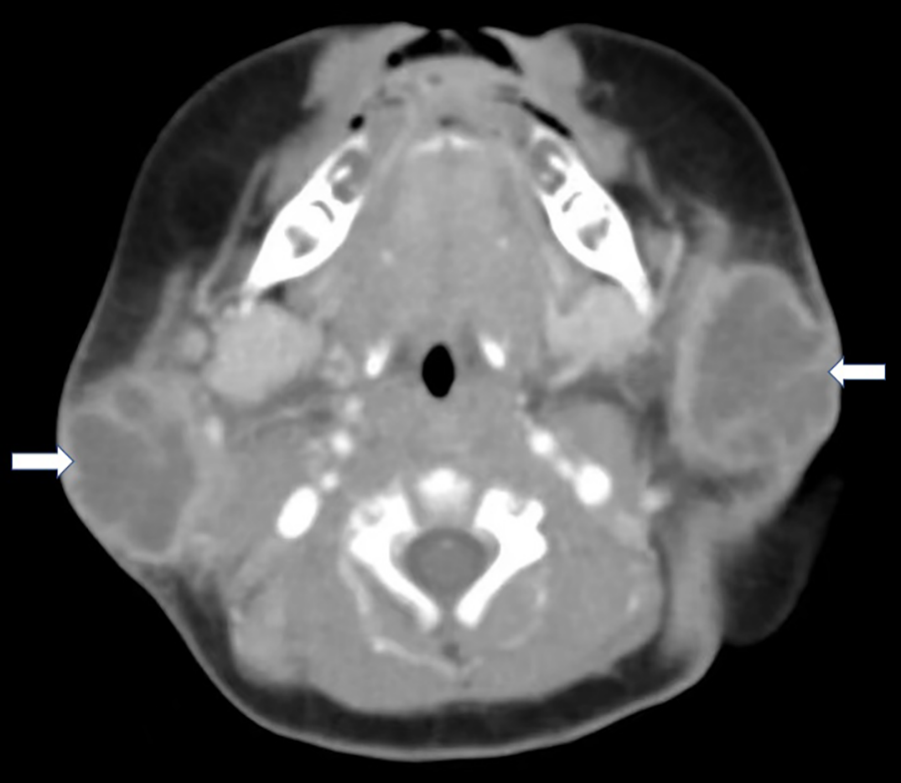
Axial contrast-enhanced CT found multiloculated low attenuation masses with rim enhancement in the bilateral parotid gland (white arrow).
